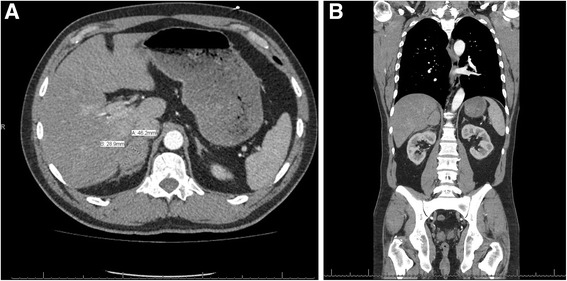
a Initial computed tomography scan at admission: right adrenal gland with an ovoid collection fluid consistent with blood measuring 4.6 × 2.9 cm in size with periadrenal stranding and with blood tracking along the inferior margin of the right hepatic lobe. b Coronal view of initial computed tomography at admission
